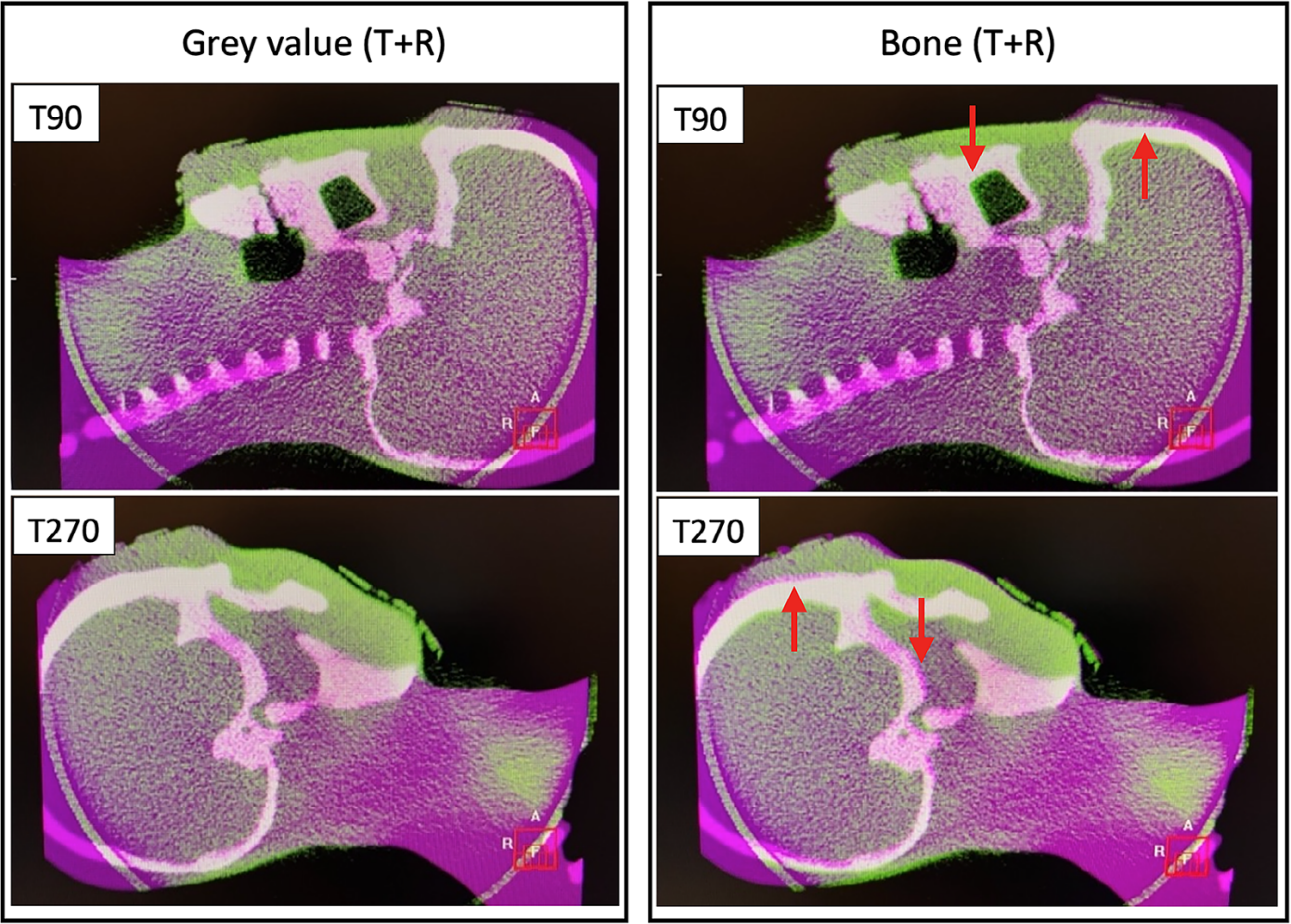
CBCT‐CT registration images with bone (T+R) mode registration method and gray value (T+R) mode registration method at turntable angles of 90° and 270°; the red arrows indicate the misalignments of bony structures. CBCT, cone‐beam CT.
